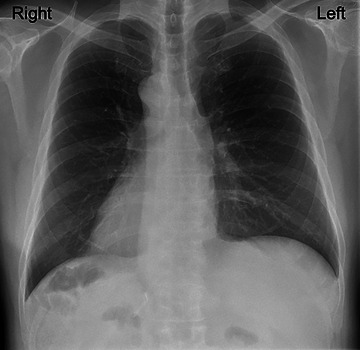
Chest radiograph demonstrating situs inversus totalis. Chest radiography demonstrated dextrocardia and a mirror‐image arrangement of the thoracoabdominal organs.
